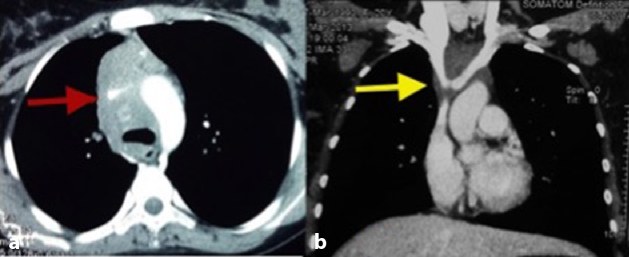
A contrast-enhanced CT scan of the chest. Axial image in the mediastinal window (a) shows an ill-defined, hypodense mass causing attenuated calibre of the superior vena cava (arrow) and narrowing of the proximal segments of the brachiocephalic veins (arrow) in the coronal image (b).
